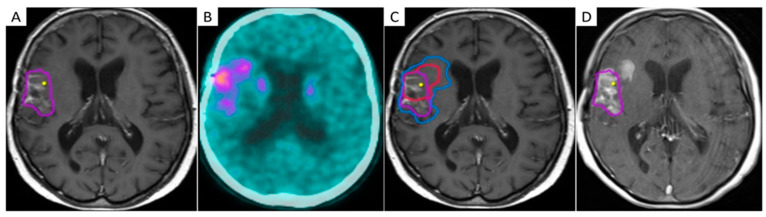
Integration of F-DOPA PET/CT in a LINAC-based radiosurgical planning of a 55-year-old female patient with recurrent GBM. (A) shows GTV based on contrast-enhanced T1-weighted MRI images; (B) shows remarkable F-DOPA uptake extending beyond contrast-enhancement. The final target volume was delineated by taking into account the F-DOPA-positive signal (C). Three-month follow-up (D) shows tumor progression at the site of pre-RT increased F-DOPA uptake, despite salvage irradiation.
